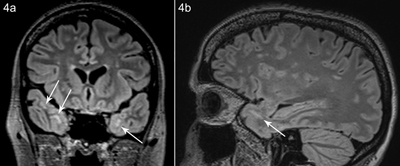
Patient B, coronal (a) and sagittal (b) FLAIR image shows white matter hyperintensity subcortical anteromedial in the temporal lobe.
